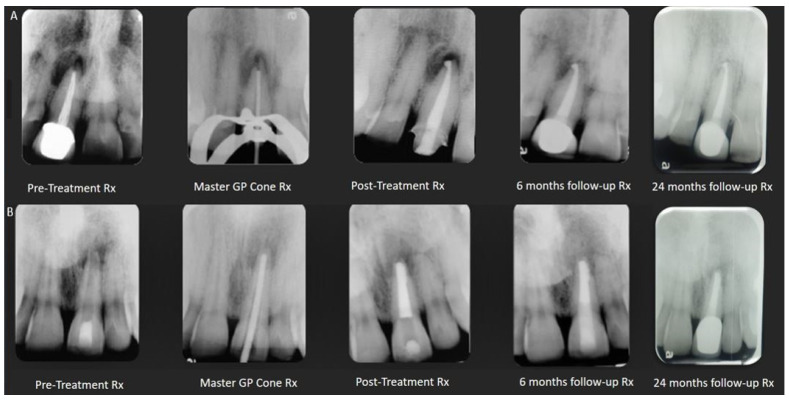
(A,B): Maxillary anterior teeth with non-divergent open apices that were obturated with GP and TSS. X-ray images of pre-treatment, GP master placement, post-treatment, and from 6-month and two-year follow-up sessions [71].
